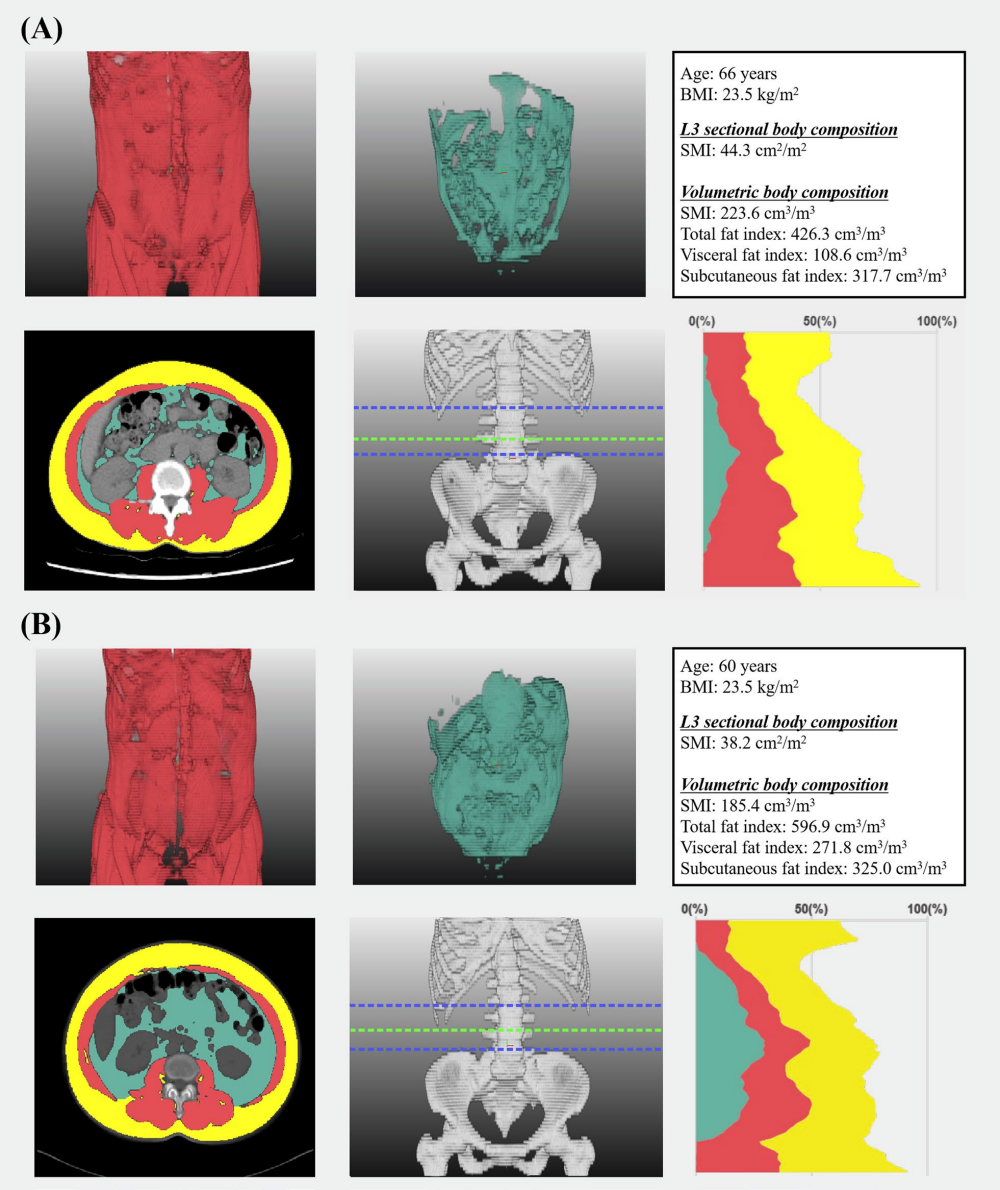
Evaluation of body composition using CT image. Despite similar baseline BMI, two patients showed different body composition profiles as follows: A a 66-year-old, non-sarcopenic woman with high-volumetric SMI; B a 60-year-old, sarcopenic woman with low-volumetric SMI. Red, skeletal muscle; green, abdominal visceral fat; yellow, subcutaneous fat; blue lines indicate the waist; light green line indicates L3 level. L3 level cross-sectional images are also presented
